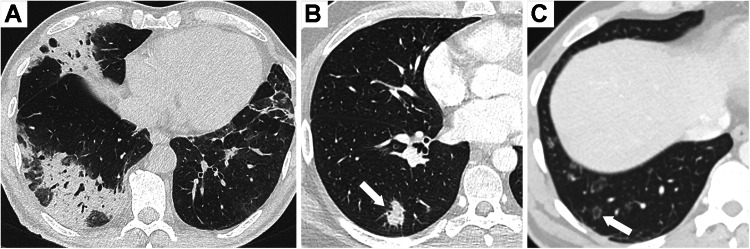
Various CT patterns of inflammatory bowel disease-related organizing pneumonia. A CT image in the axial plane shows bilateral consolidation with air bronchogram in a 71-year-old patient with Crohn’s disease. B CT image in the axial plane shows a pseudo-tumoral lesion (arrow) in a 36-year-old patient with Crohn’s disease. C CT image in the axial plane shows multiple consolidations with the atoll sign (arrow) in a 35-year-old patient with Crohn’s disease
